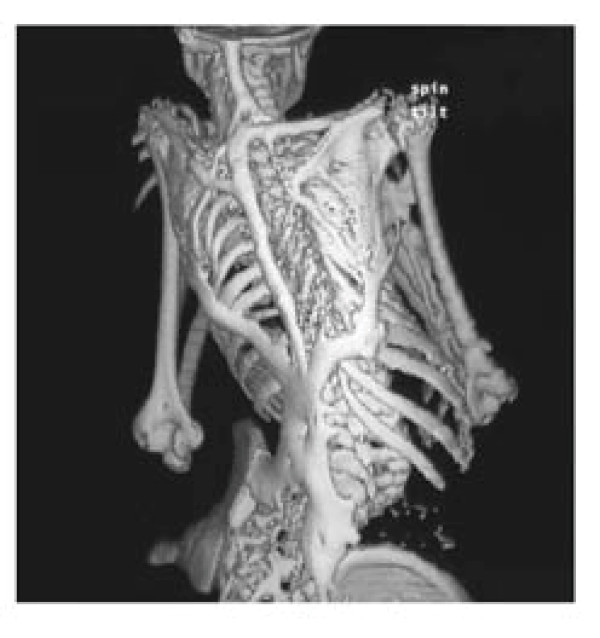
Three-dimensional reconstructed computed tomography (CT) scan of the back of a twelve-year old child showing extensive heterotopic ossification typical of FOP. Reprinted from reference 14. Authors retain copyright.
